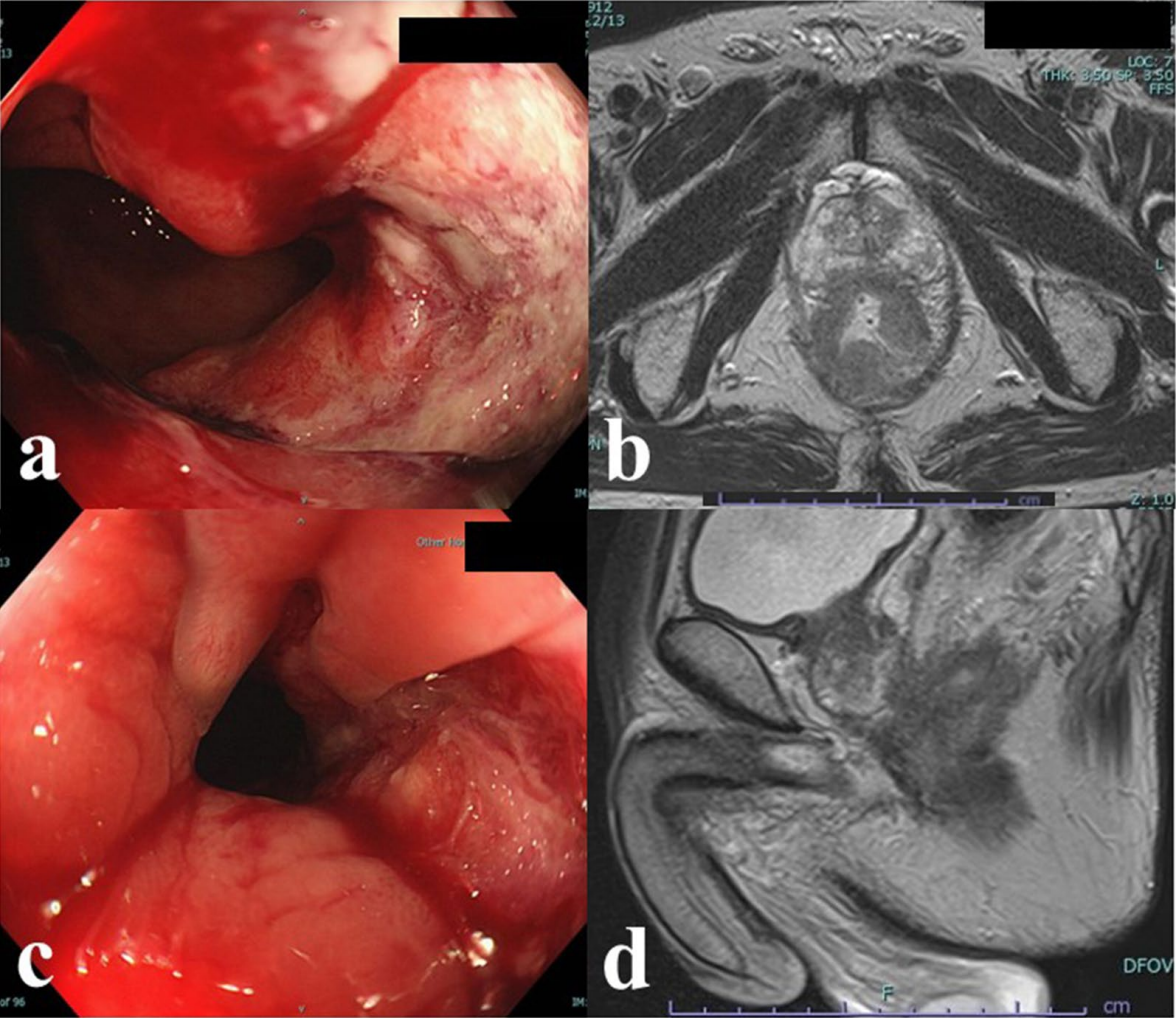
a Colonoscopy image and b magnetic resonance image at the time of the initial diagnosis. The ulcerative tumor is located in the lower rectum and proctodeum. Biopsy revealed tubular adenocarcinoma. c Colonoscopy image and d magnetic resonance image after neoadjuvant chemoradiotherapy. Partial response was observed. However, the prostate was still involved
